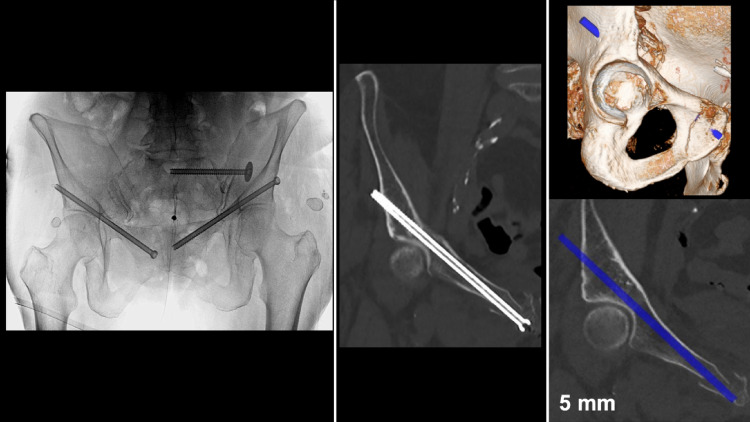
Same patient as in Fig. 1. On the left, anteroposterior fluoroscopic view demonstrating bilateral superior pubic ramus screws accurately positioned. In the center, postoperative CT scan showing the screw located within the intramedullary corridor at a safe distance from the acetabular joint, without cortical breach. On the right, CT-based planning using a 5-mm cylindrical model corresponding to the screw core diameter, demonstrating a feasible intramedullary corridor despite the absence of a feasible trajectory using a 6.5-mm rectilinear model
